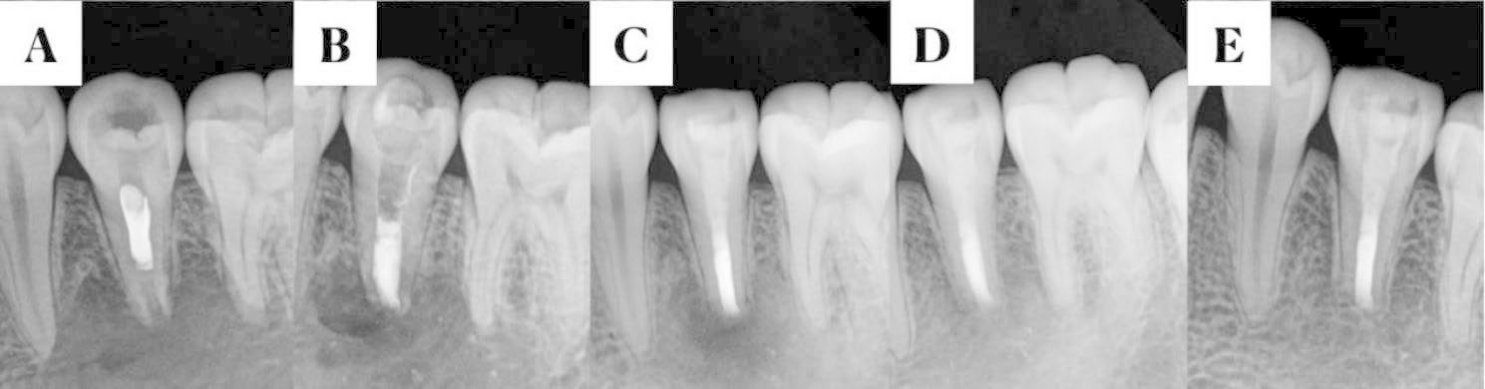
Changes in the periapical tissue radiographs after endodontic microsurgery upon revascularization failure. (A) Preoperative radiograph, (B) postoperative radiograph, and (C) follow-up radiographs after 3 months, (D) 6 months, and (E) 1 year
